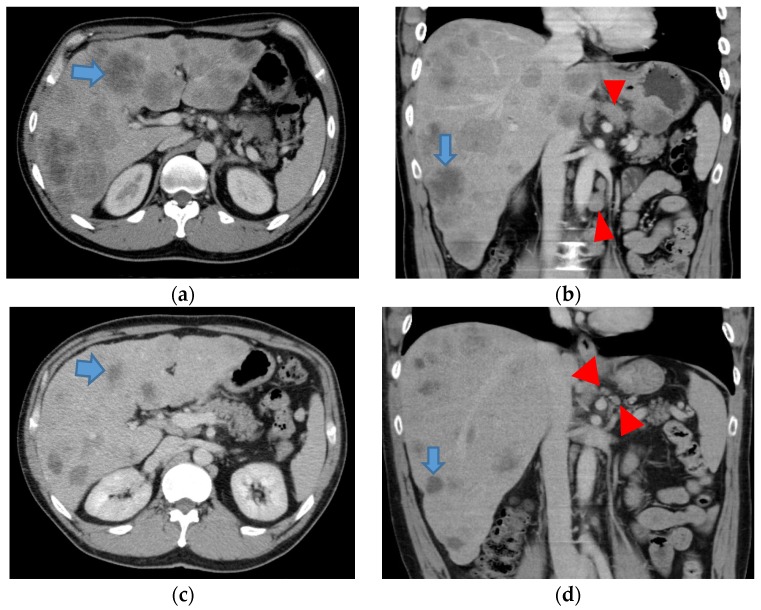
CT scan showed multiple liver tumors and swellings of lymph nodes before the start of chemo-immunotherapy with trastuzumab (a,b). Liver tumors (blue arrows) and lymph nodes (red arrow heads) remarkably decreased in size after four cycles of treatment (c,d).
